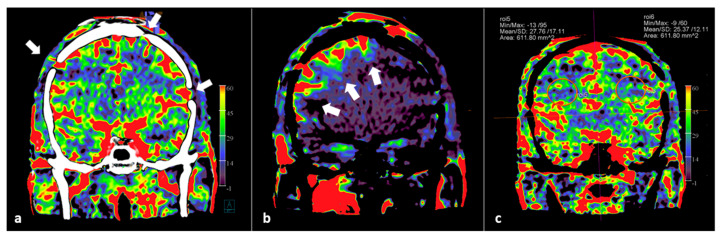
Selective and whole brain FD CT PBV imaging in a 29-year-old Asian male with moyamoya disease, treated by multiple burr holes surgery. Scanning was performed during aortic root injection (whole brain PBV imaging) and during selective ECA injection. (a) Coronal mask and PBV fusion MIP reformation demonstrates symmetrical PBV maps, and shows that multiple burr holes are present (white arrows). (b) Coronal PBV MIP reformation with selective right ECA contrast injection displays the blood supply to the right cerebral hemisphere (white arrows). (c) PBV value measurements in symmetrically plotted ellipsoid regions of interest (ROI) show symmetric PBV values.
